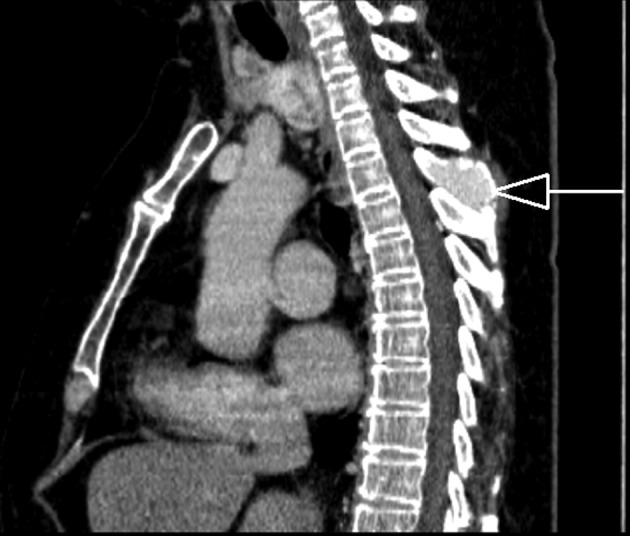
Computed tomography with contrast showing the 4th thoracic vertebra with bony destruction, and an enhanced lesion.
